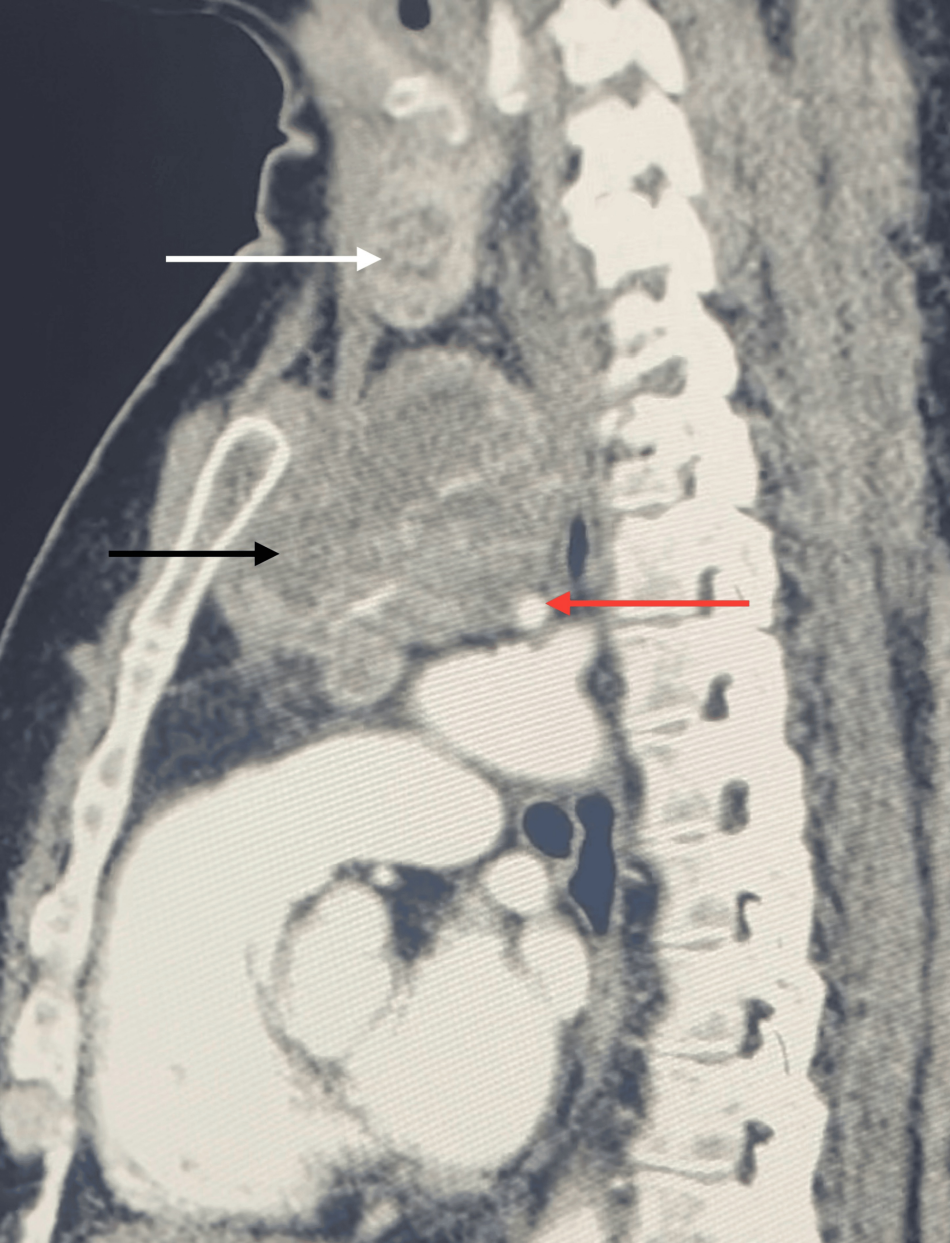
CT scan showing the EMG (black arrow) with its mediastinal blood supply (red arrow), separated from the thyroid gland (white arrow) EMG, ectopic mediastinal goiter
